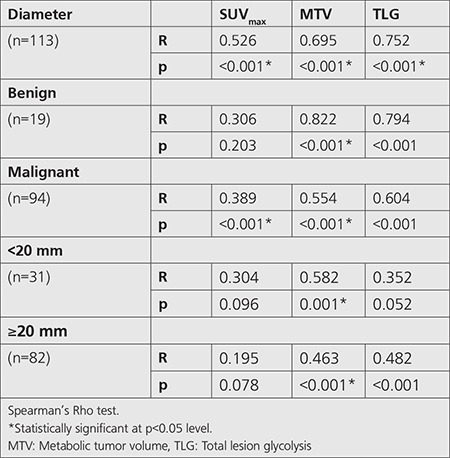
Relationship between ^18^F-FDG positron emission tomography/computed tomography SUV_max_ and volumetric parameters and diameter
